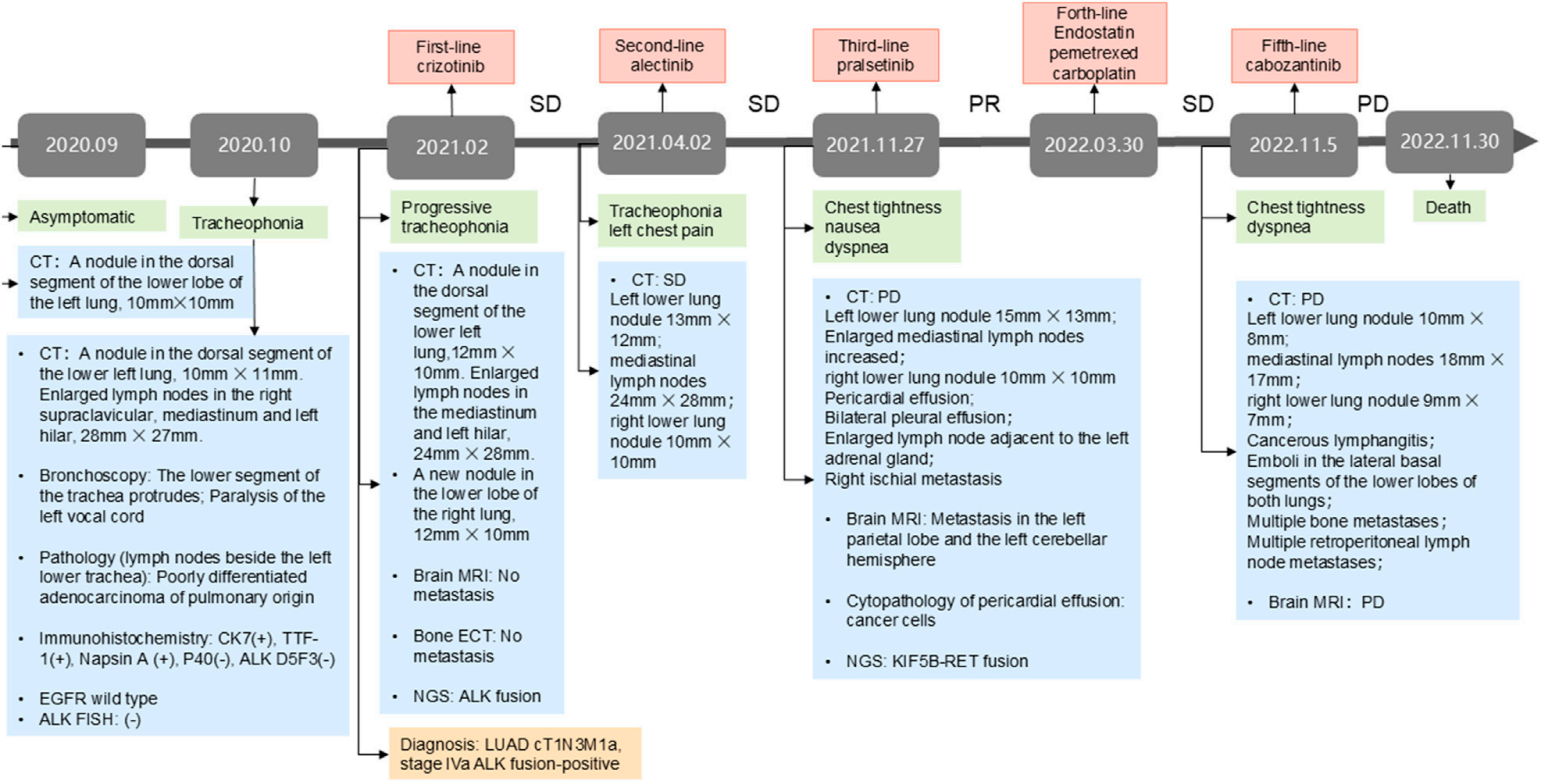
The treatment milestone of the patient. NGS, next-generation sequencing; PR, partial response; SD, stable disease; PD, progressive disease; TMB, tumor mutational burden; LUAD, lung adenocarcinoma. CT, computed tomography; MRI: magnetic resonance imaging.
